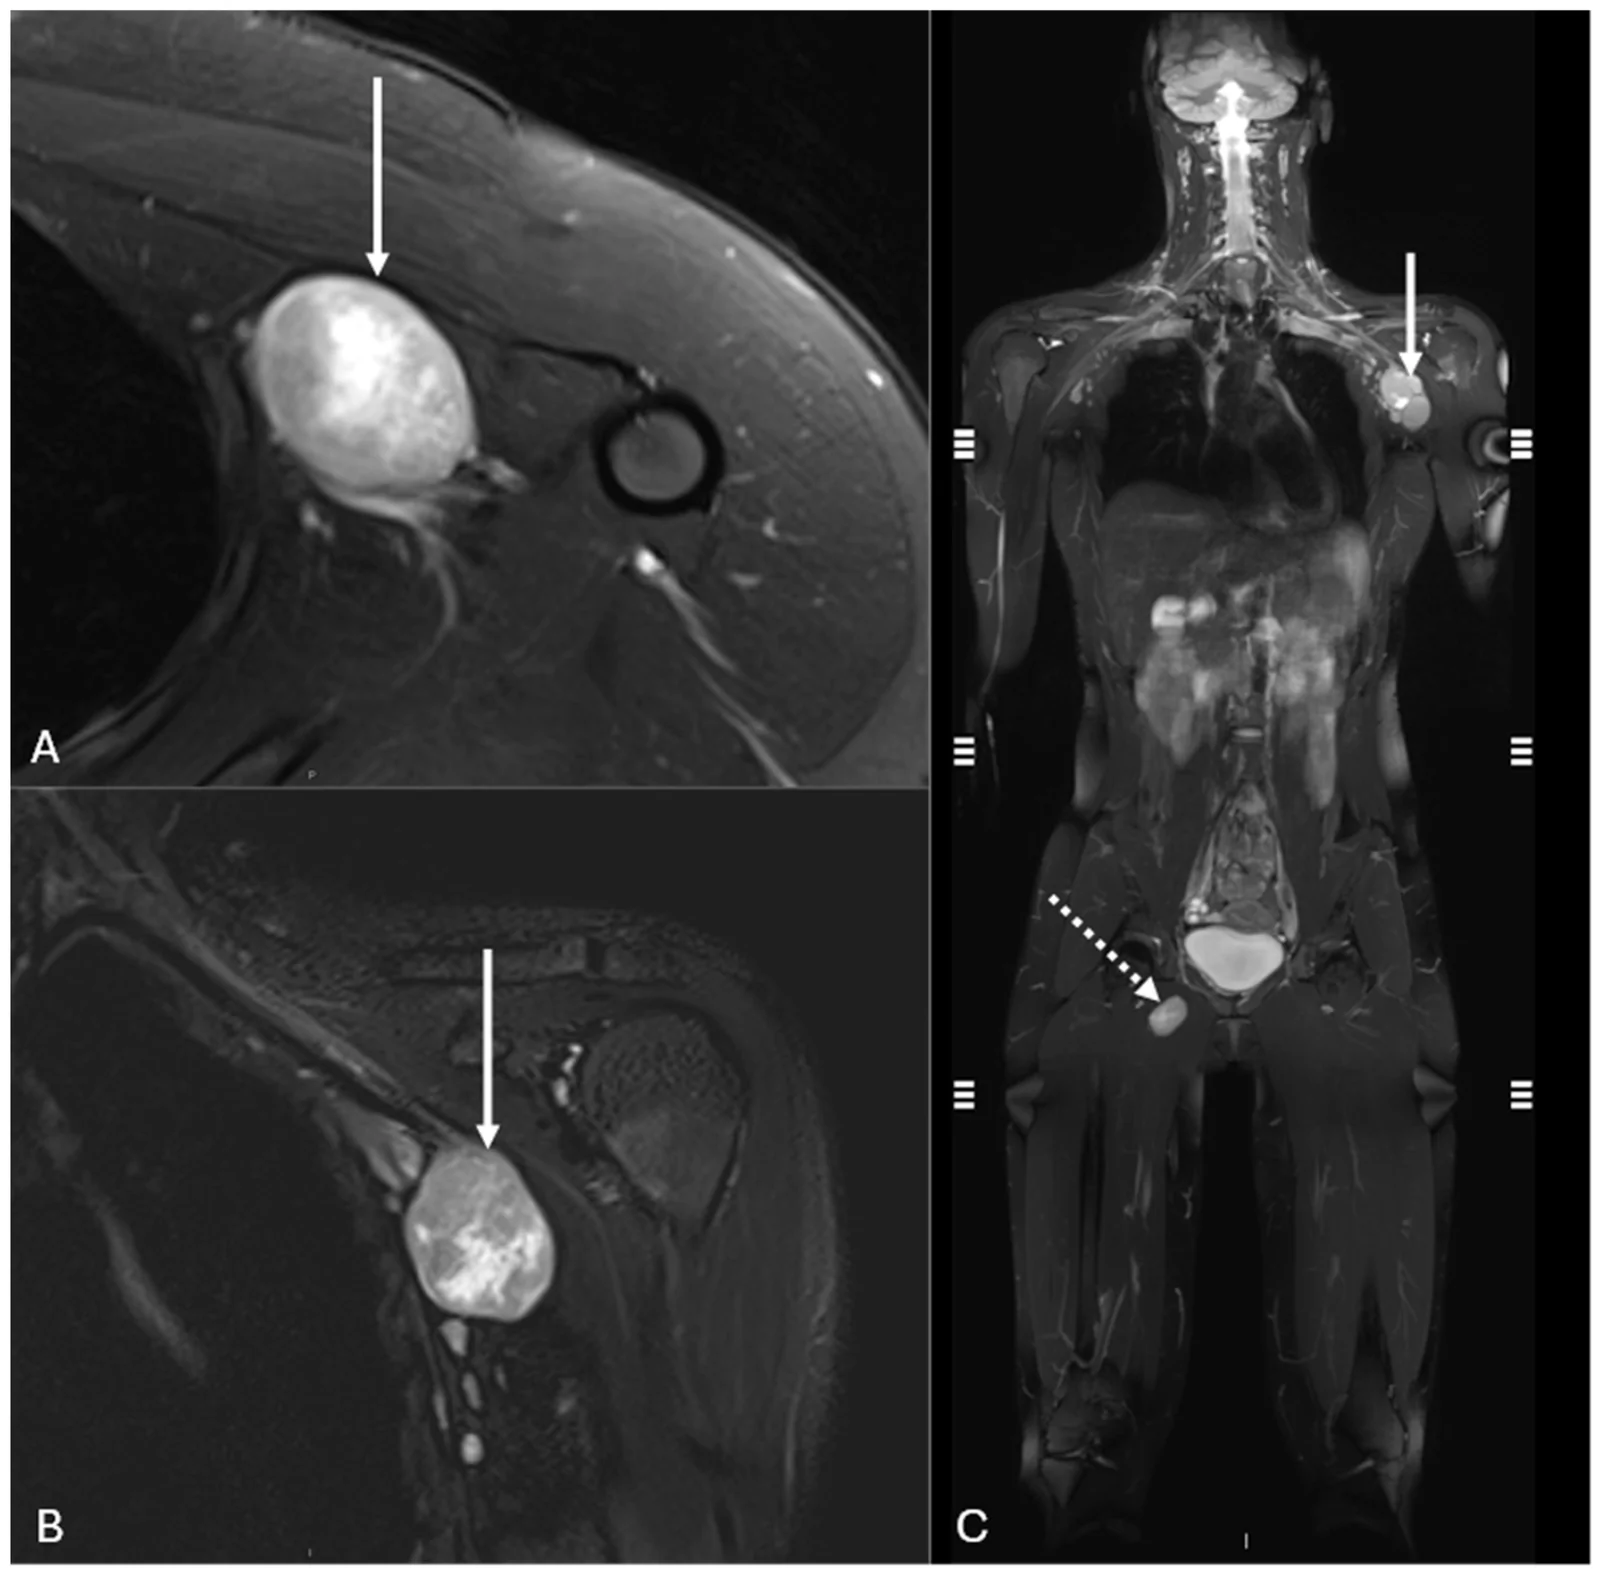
15-year-old female with positive family history for SWN-NEC and one pathologically proven left brachial plexus schwannoma (solid arrow on (A–C)) status post debulking. Whole-body MRI detected an additional right medial thigh presumed schwannoma (dashed arrow on (C)). Genetic testing did not detect a known pathogenic variant associated with SWN.
